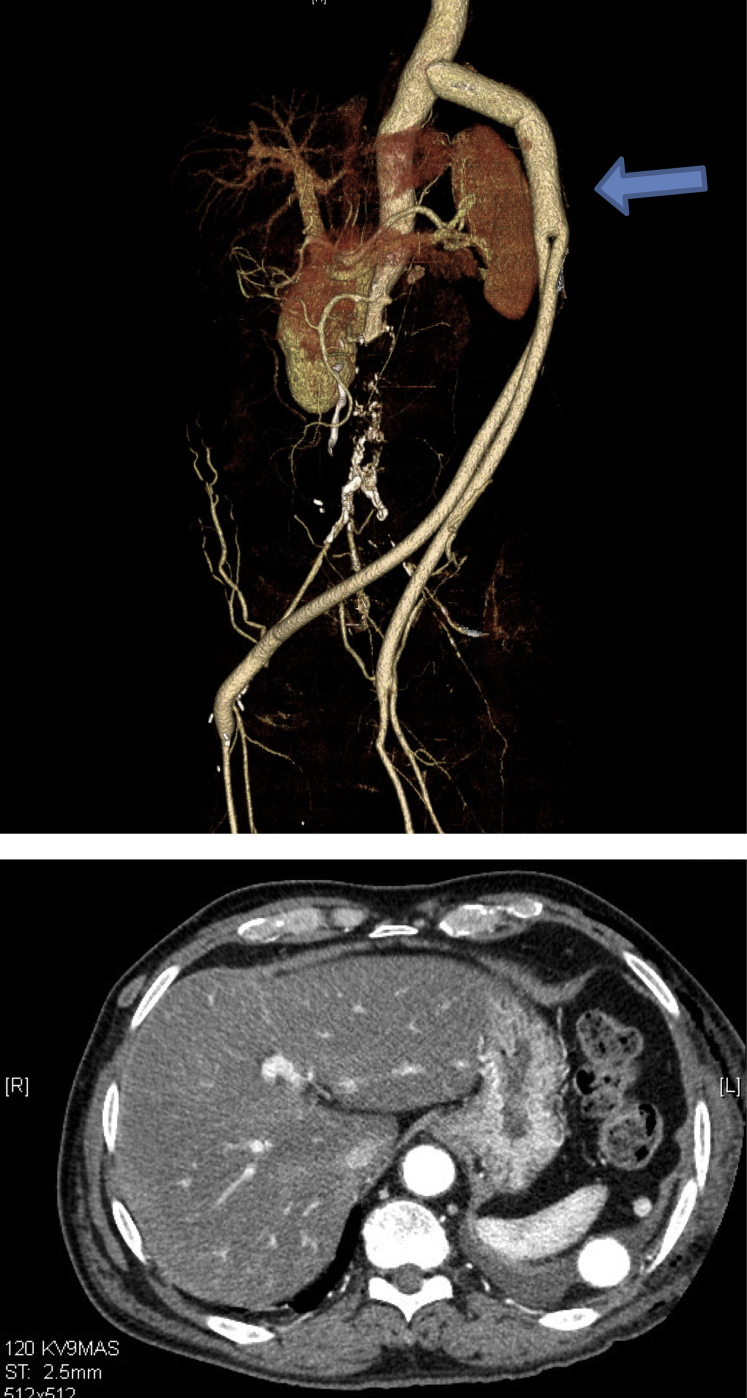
Computed tomographic angiography after thoracobifemoral bypass grafting including vascular reconstruction (top) and cross-section (bottom). Note the tunnel of the main body of the graft in the left lateral diaphragm (*arrow*).
